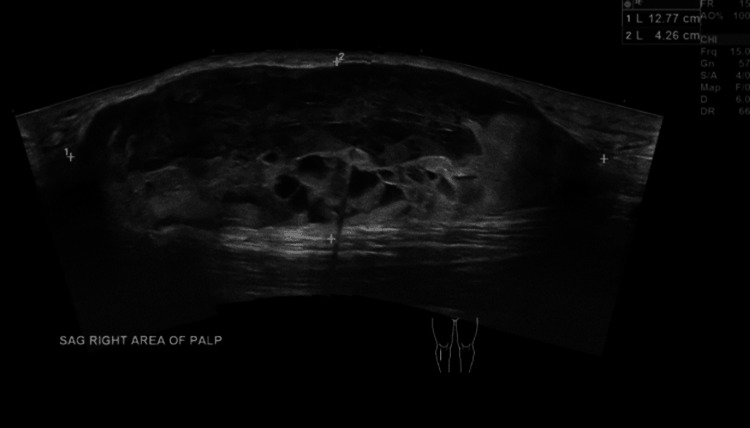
Ultrasound of the right lower extremity Two months after initial imaging. There is now a heterogeneous mass measuring 12.8 × 4.3 × 9.4 cm which appears external to the muscle fascia in the anterior right leg. The size has increased since the prior MRI.
